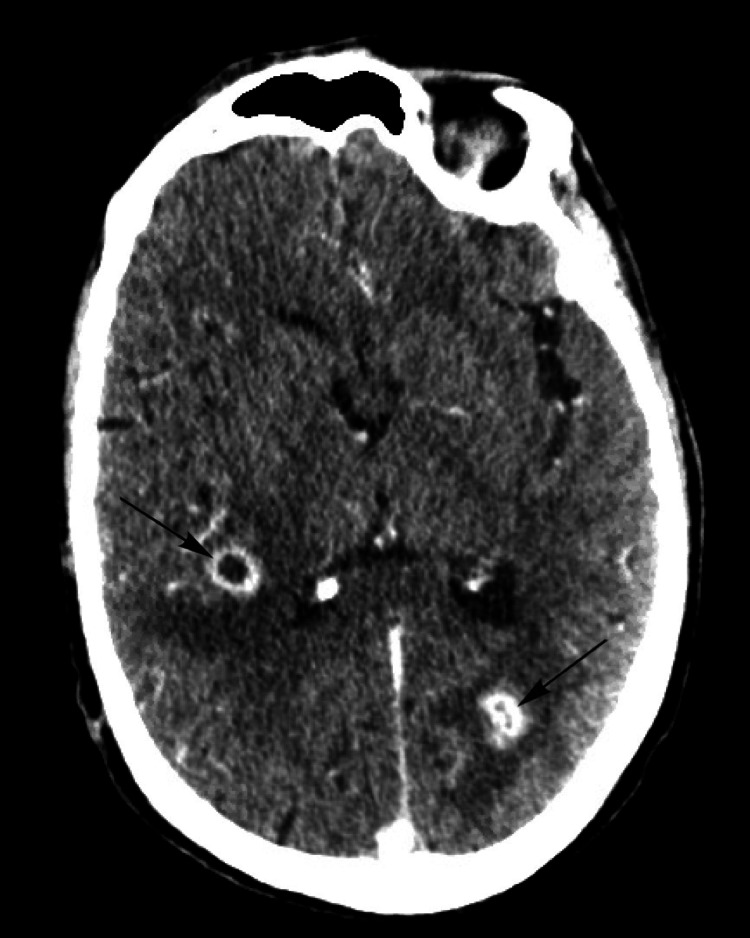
CT head with intravenous contrast showing two rim-enhancing lesions (arrows) in the right temporal and left parietal lobes with surrounding vasogenic edema. CT: computed tomography
